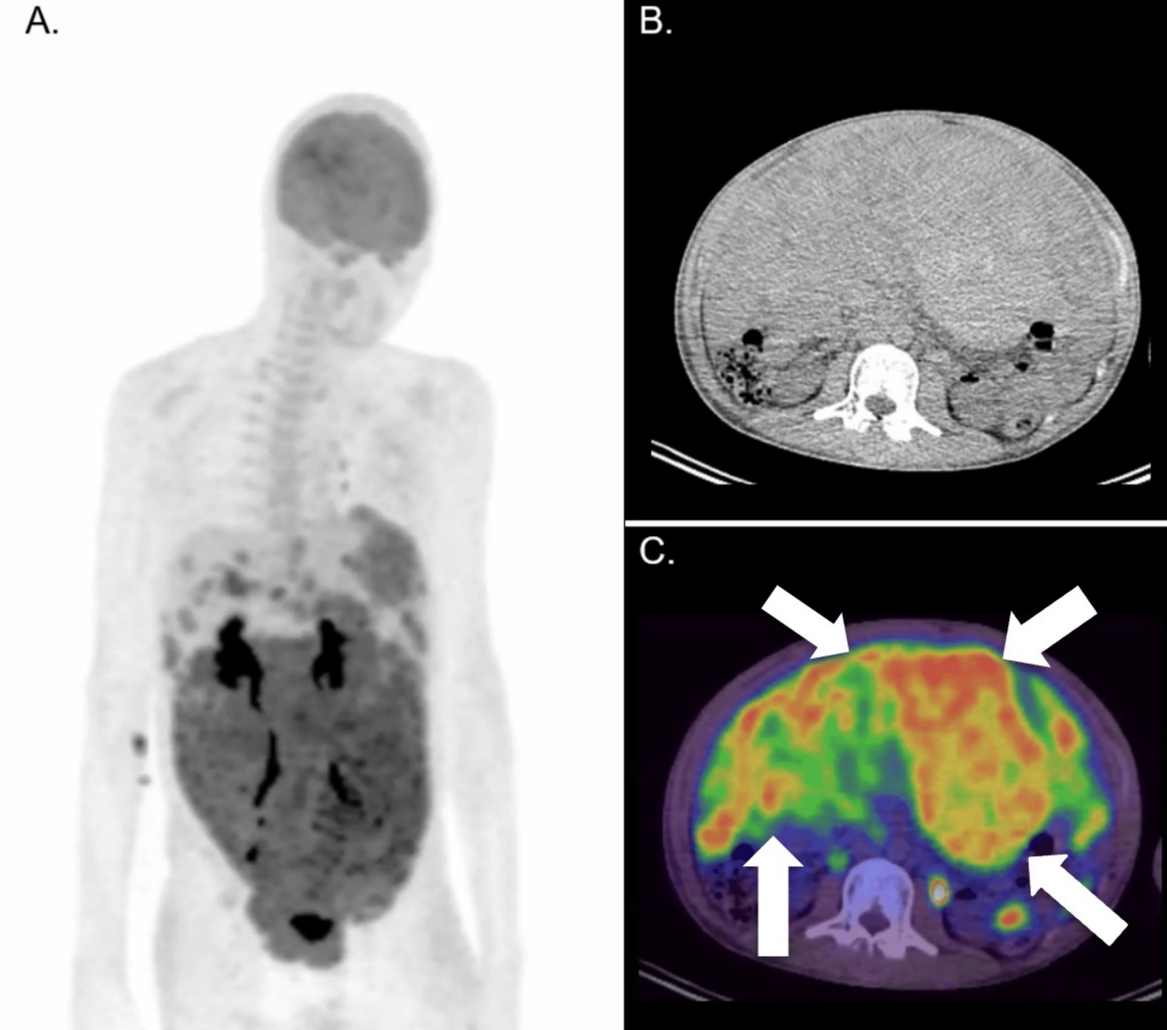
Pre-treatment 18F-FDG PET/CT scan The initial axial maximum intensity projection (MIP) (2A) and axial non-contrasted CT (2B) demonstrated extensive abdominal and pelvic masses, primarily involving the peritoneum and encasing most of the bowel loops. Fused PET/CT images (2C) highlighted intense FDG uptake in these masses, reflecting the high metabolic activity typical of DSRCT. The peritoneal and hepatic involvement was extensive (arrows), suggesting a significant tumor burden.
